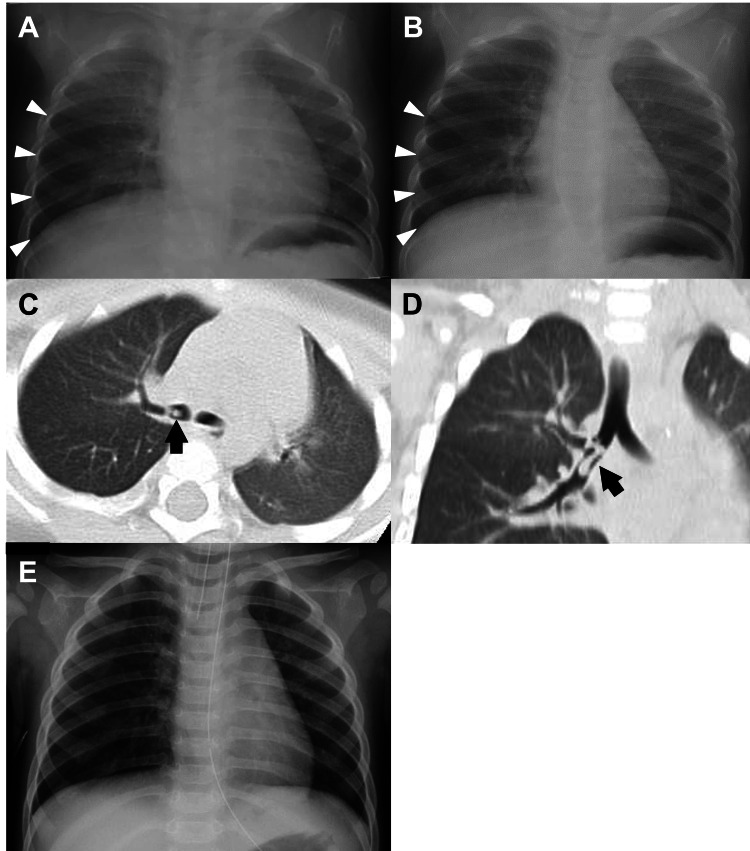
CXR showing slight emphysema of the right lower lung. (A) Expiratory phase. (B) Inspiratory phase. (C) Axial CT showing a plastic foreign body at the right inferior lobar bronchus (black arrow). (D) Coronal CT showing the seven-shaped foreign body (black arrow). (E) CXR, after removing the foreign body, in which partial emphysema disappeared. CXR, chest X-ray; CT, computed tomography
